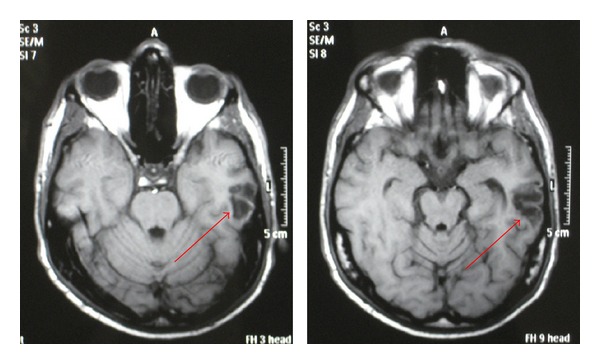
Brain MRI examination (axial T1-weighted sequence). The arrows point to an hypointensity area mainly involving left temporal subcortical white substance. It is likely to be an ischemic lesion with partial hemorrhage. Day 1 after diving session.
